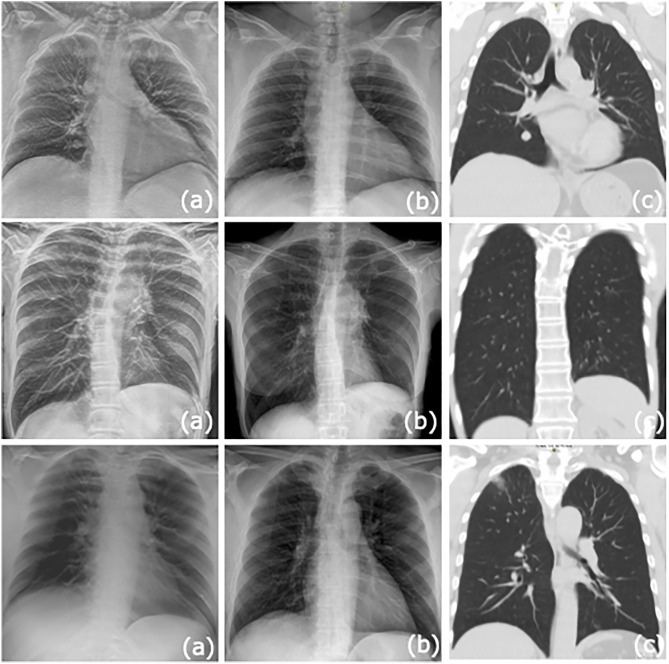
Comparison of single slices of s-DCT (a) with corresponding CXR (b). Comparison single coronal slice CT images are also provided (c). Row 1: Improved visualization of a 1.4 cm solid pulmonary nodule in the medial right middle lobe. The overlying right atrium limits evaluation on the CXR. Row 2: S-DCT and CXR show a dense left perihilar consolidation. Tomosynthesis demonstrates ground glass opacities in the retrocardiac left lower lobe, not seen on CXR, which are confirmed on chest CT. Row 3: S-DCT demonstrates a ground glass nodular opacity in the subpleural right upper lobe. Not well seen on CXR due to the overlying osseous structures. Findings are confirmed on chest CT.
